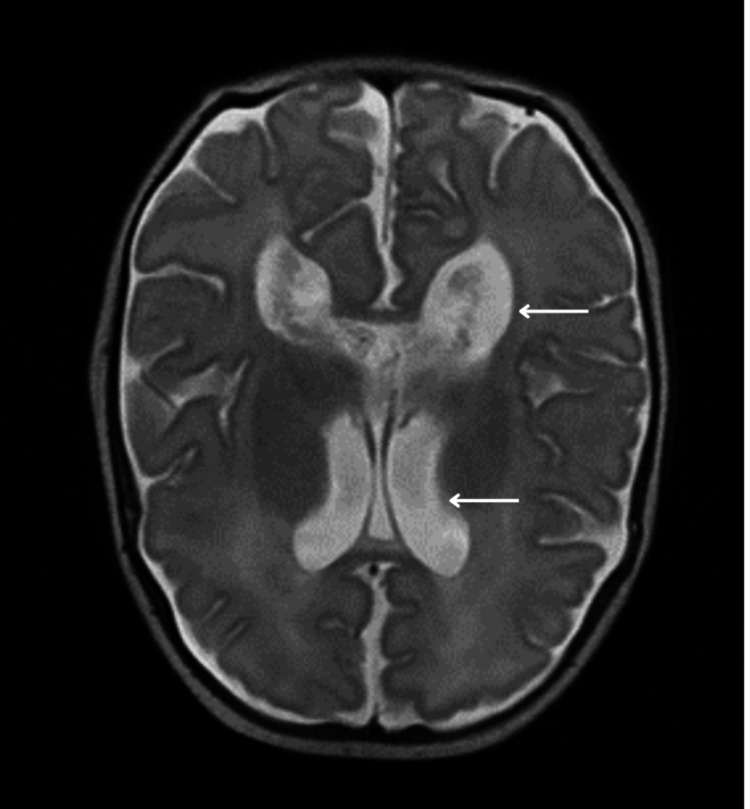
MRI brain axial T2-weighted MRI brain image that demonstrates mild dilatation of the lateral ventricles.
